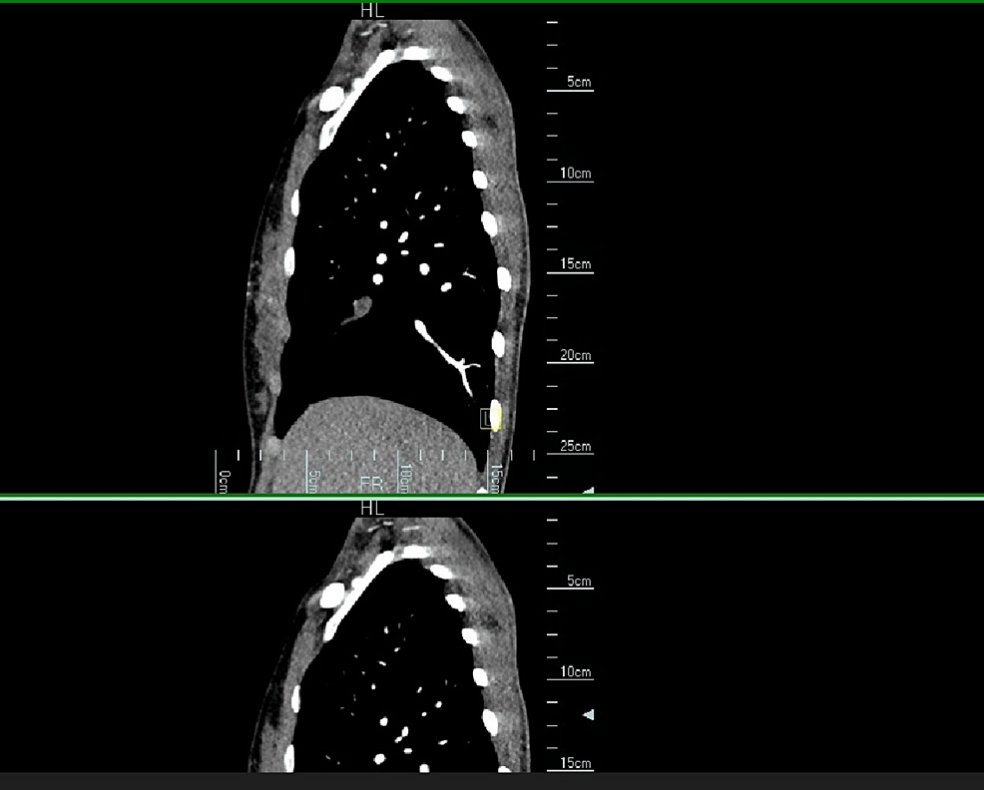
CTA sagittal view of a 28-year-old female showing a prominent tubular vessel that shows less enhancement than the opacified pulmonary artery and pulmonary veins consistent with an aberrant arterial supply of pulmonary sequestration CTA: computed tomography angiogram
